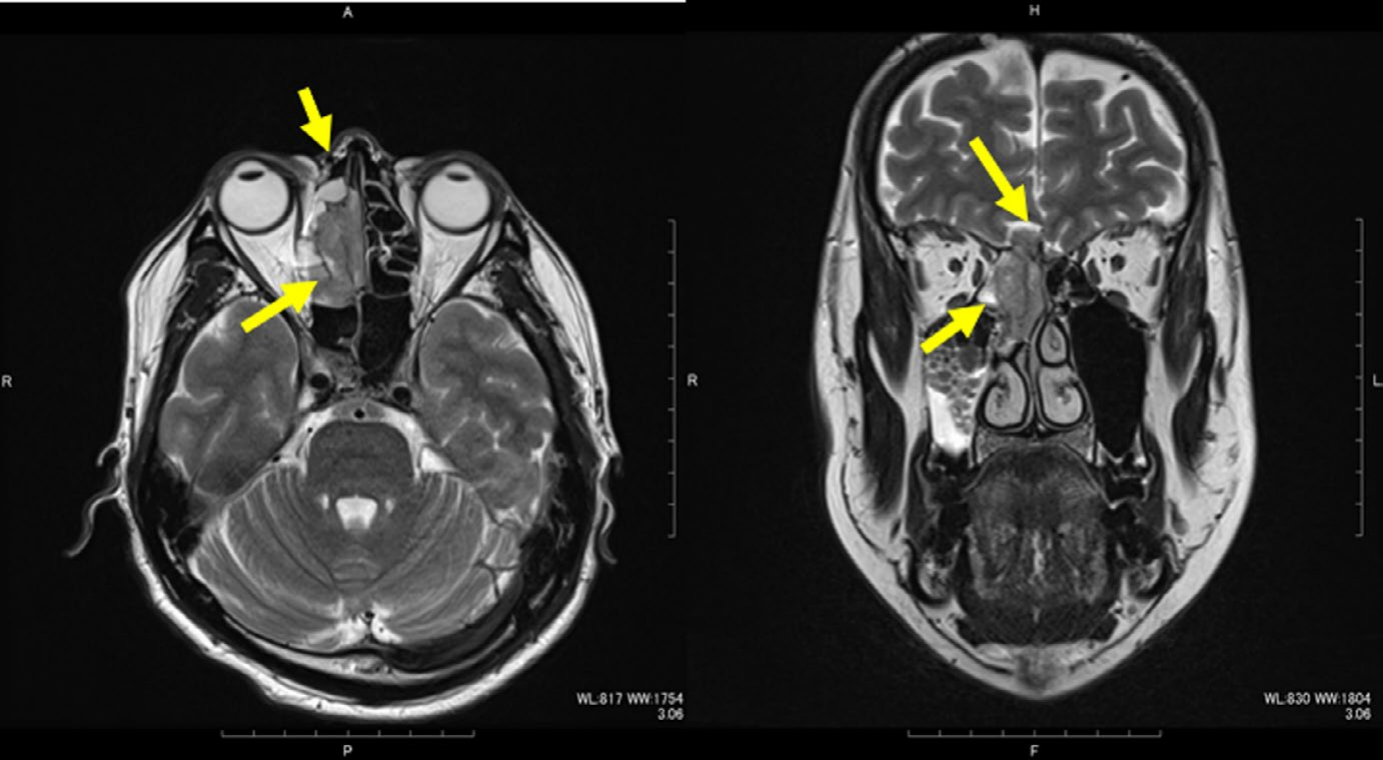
MRI findings. Left: Ethmoid sinus tumor seen attached to the right medial orbital wall but not invading the anterior aspect. Right: Tumor is attached to the right medial orbital and invaded to intracranial space
